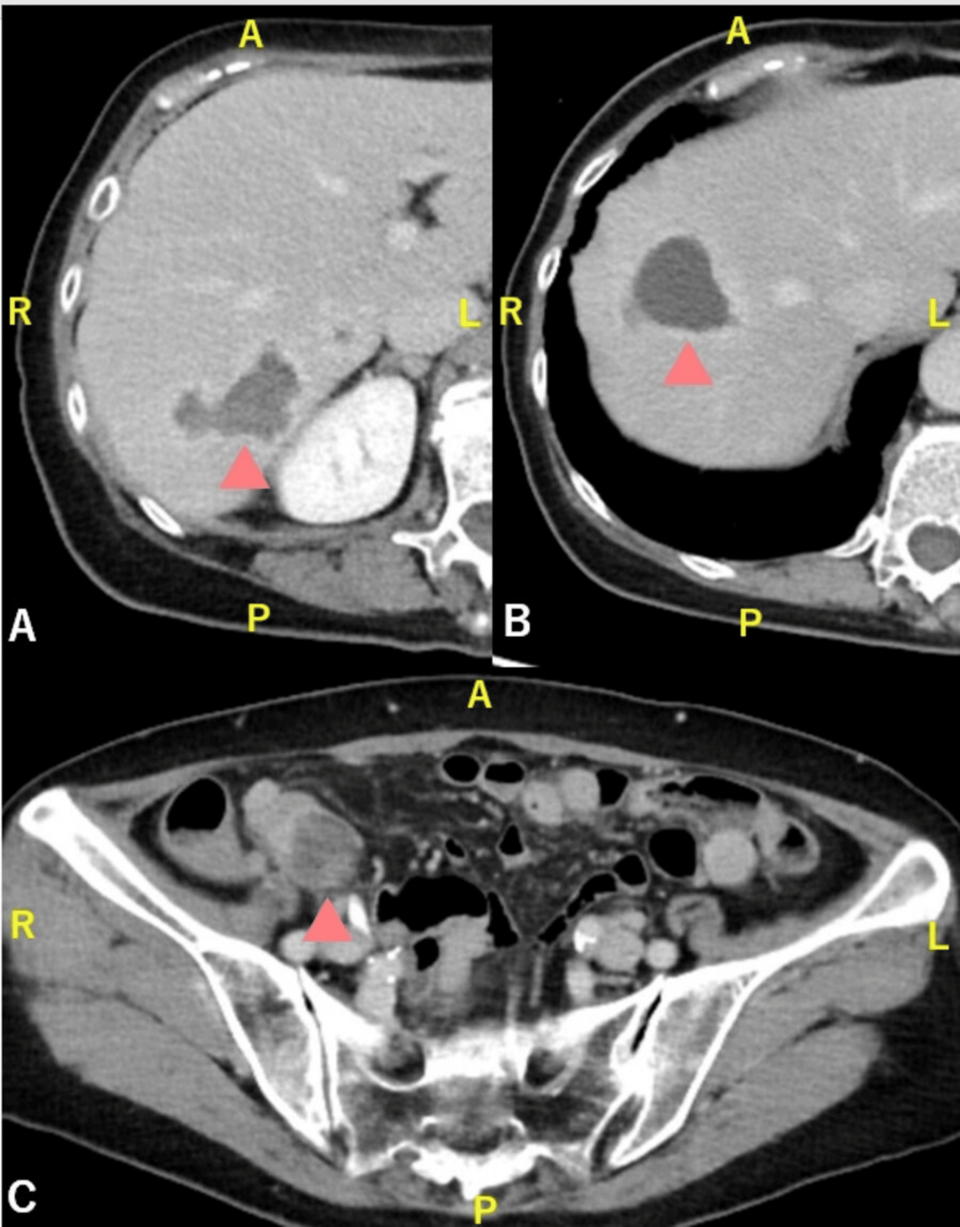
Follow-up contrast-enhanced CT after meropenem therapy and ultrasound-guided drainage of the hepatic abscesses (A, B) No remarkable improvement is observed in the liver abscesses (arrowheads). (C) The abscess adjacent to the terminal ileum persists (arrowhead) CT: computed tomography
